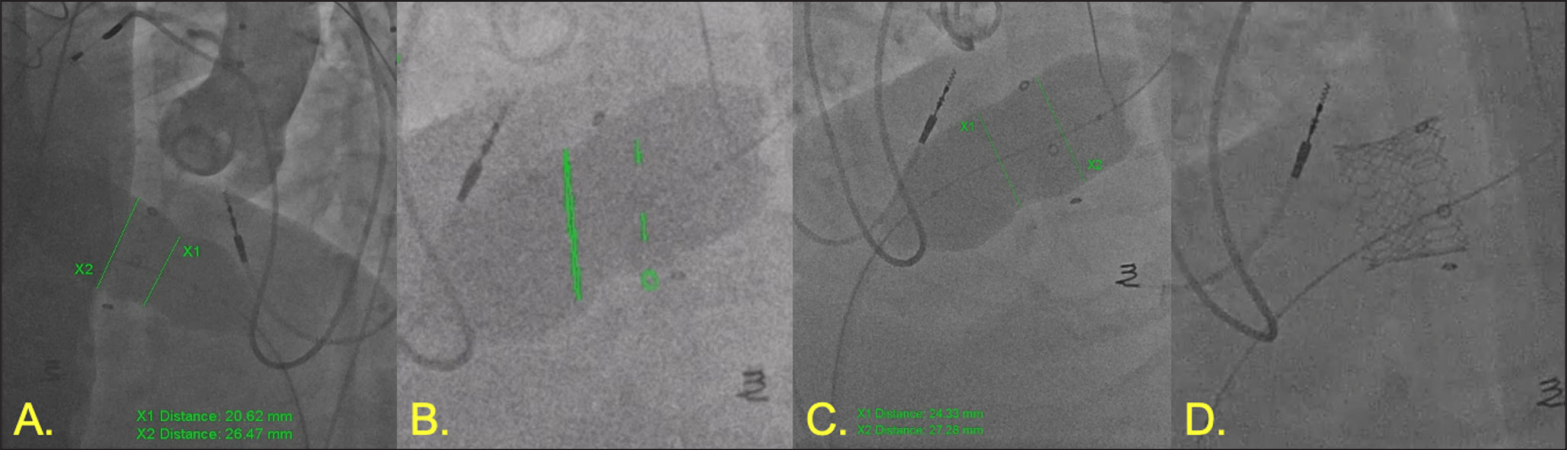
Bioprosthetic tricuspid valvuloplasty. (A) 26-mm Vida valvuloplasty balloon inflated showing 20.6 mm at waist. (B) 28-mm True balloon inflated for intentional value fracture (green markers showing landmarks created using 2D/3D registration from computed tomography angiography). (C) Post valve fracture measurement with improvement. (D) SAPIEN 3 Ultra 26-mm valve successfully deployed.
